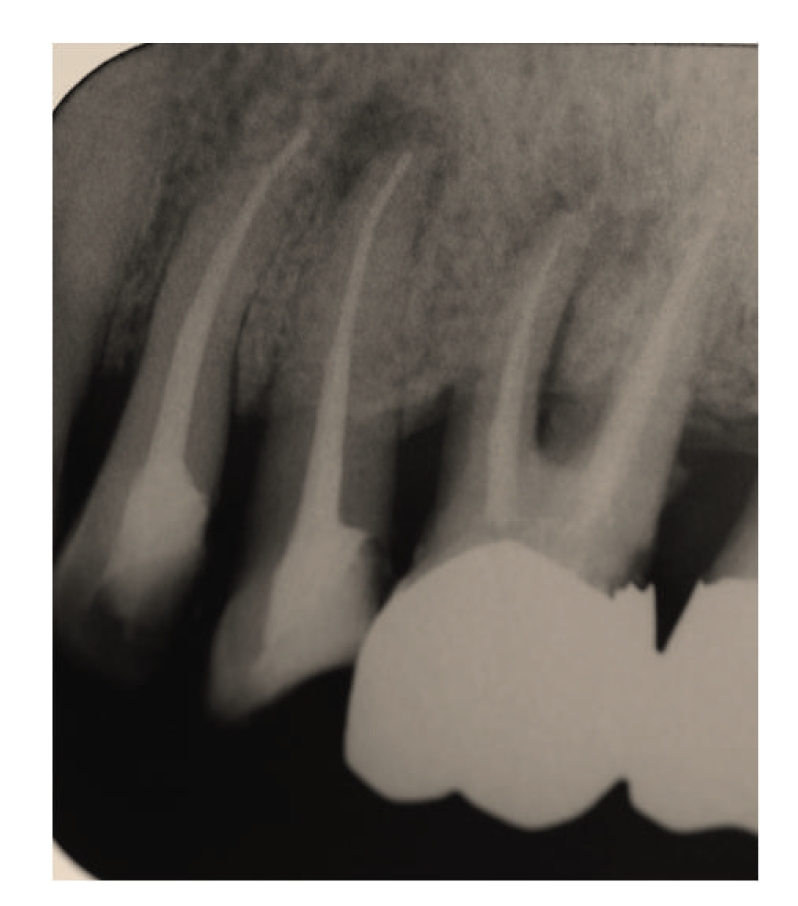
Case 1: Radiography of the root canal filling showing remaining bone loss at the root apex.
